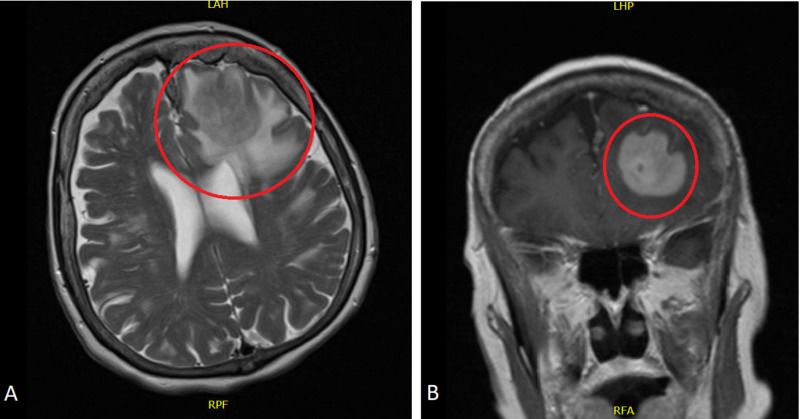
Magnetic resonance imaging of brain with contrast Horizontal (A) and coronal (B) sections of brain MRI showing a dominant left frontal mass (red circles) approximately 3.8 cm in diameter with adjacent rim of vasogenic edema MRI: magnetic resonance imaging
